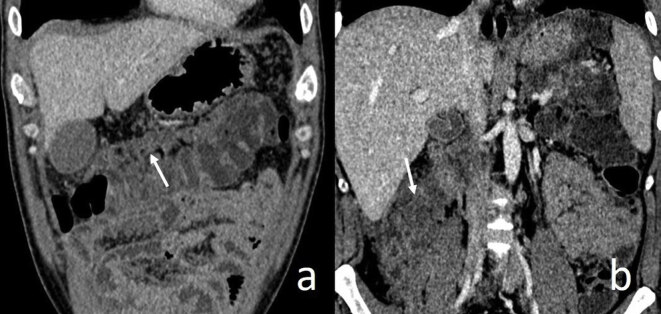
FND in mass form is seen at the transverse mesocolon resembling an omental cake (arrow) (a). Also, FND in mass form is seen close to the right psoas muscle on the coronal contrast-enhanced abdomen CT image (b).
